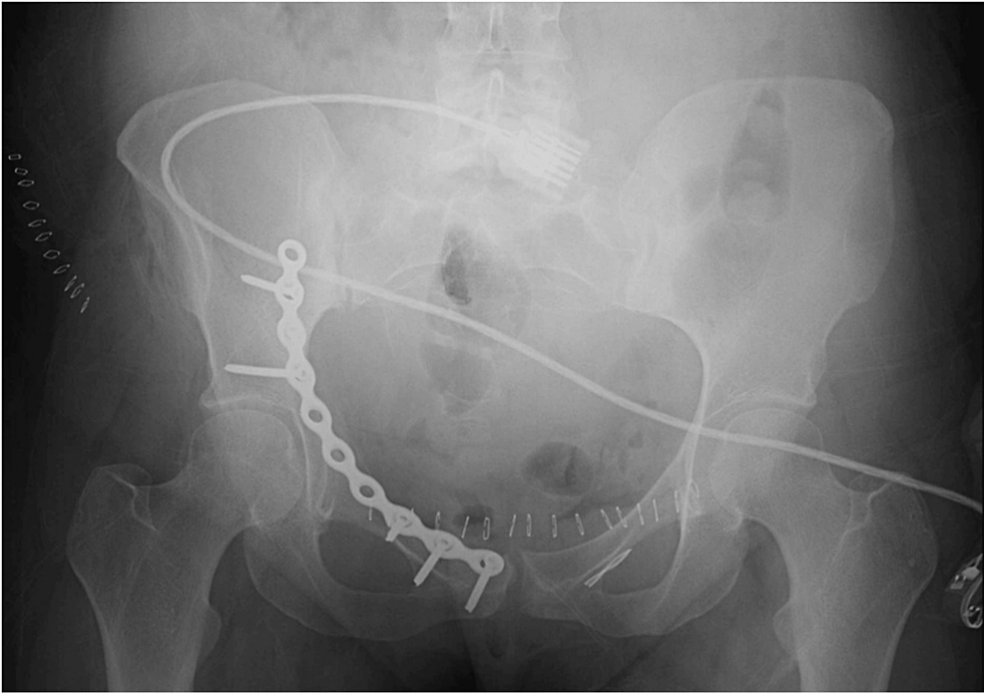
Postoperative x-ray of the pelvis showing placement of pelvic recon plate and screws.
